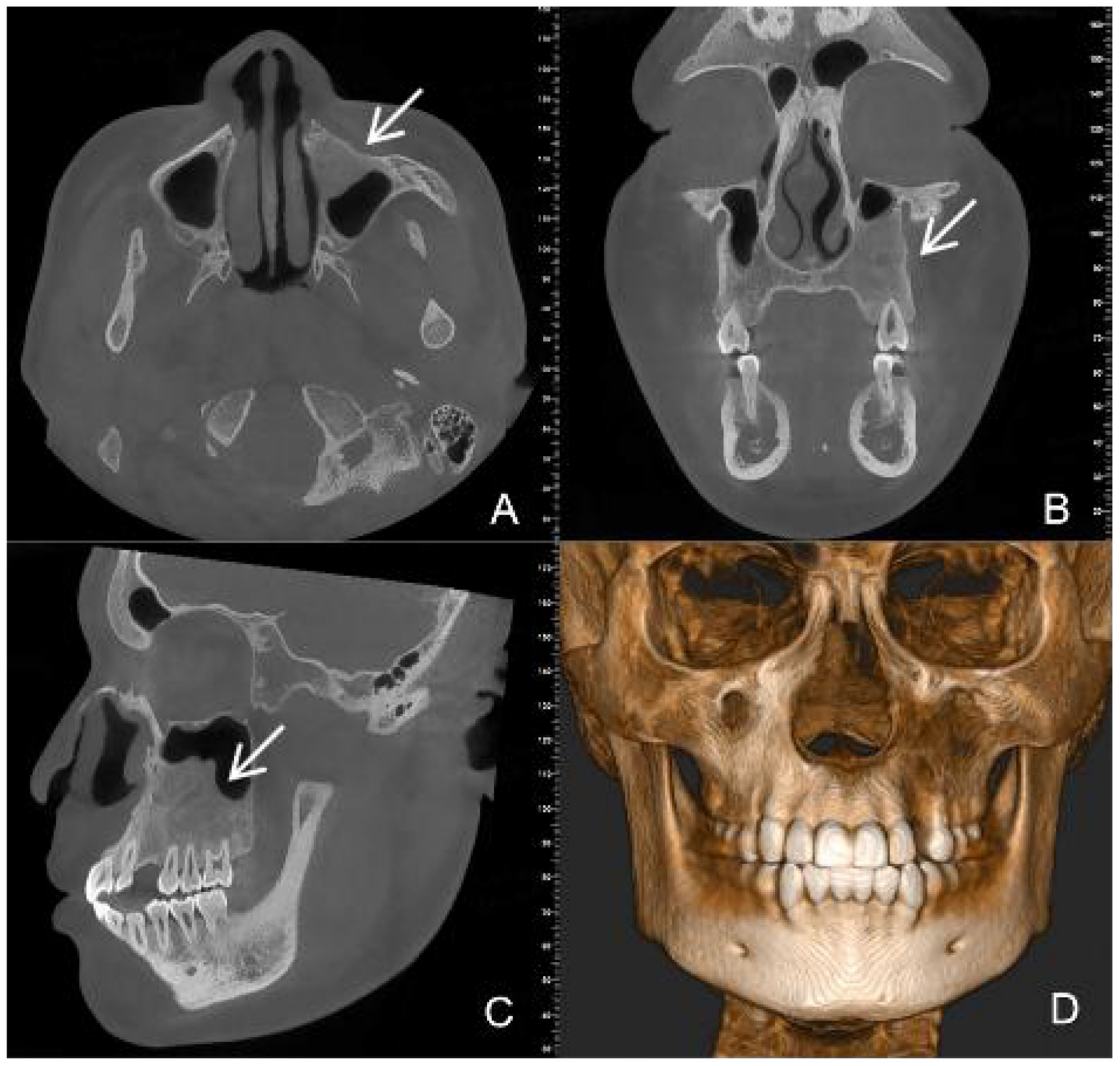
CBCT images at six months after tumour resection (A) (Axial view) (B) (Coronal view) (C) (Sagittal view) (D) (Three-dimensional reconstruction) show complete bone remodeling (white arrow).
